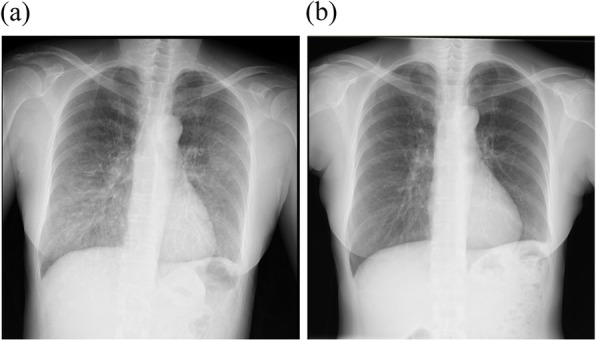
Chest roentgenogram findings. Each image has ground-glass opacity in the lower lung fields on both sides. a Image taken on June 2, 20XX, in the standing position, tube voltage of 130 kV, and tube current of 250 mA. b Image taken on June 22, 20XX, in the standing position, tube voltage of 130 kV, and current of 250 mA
